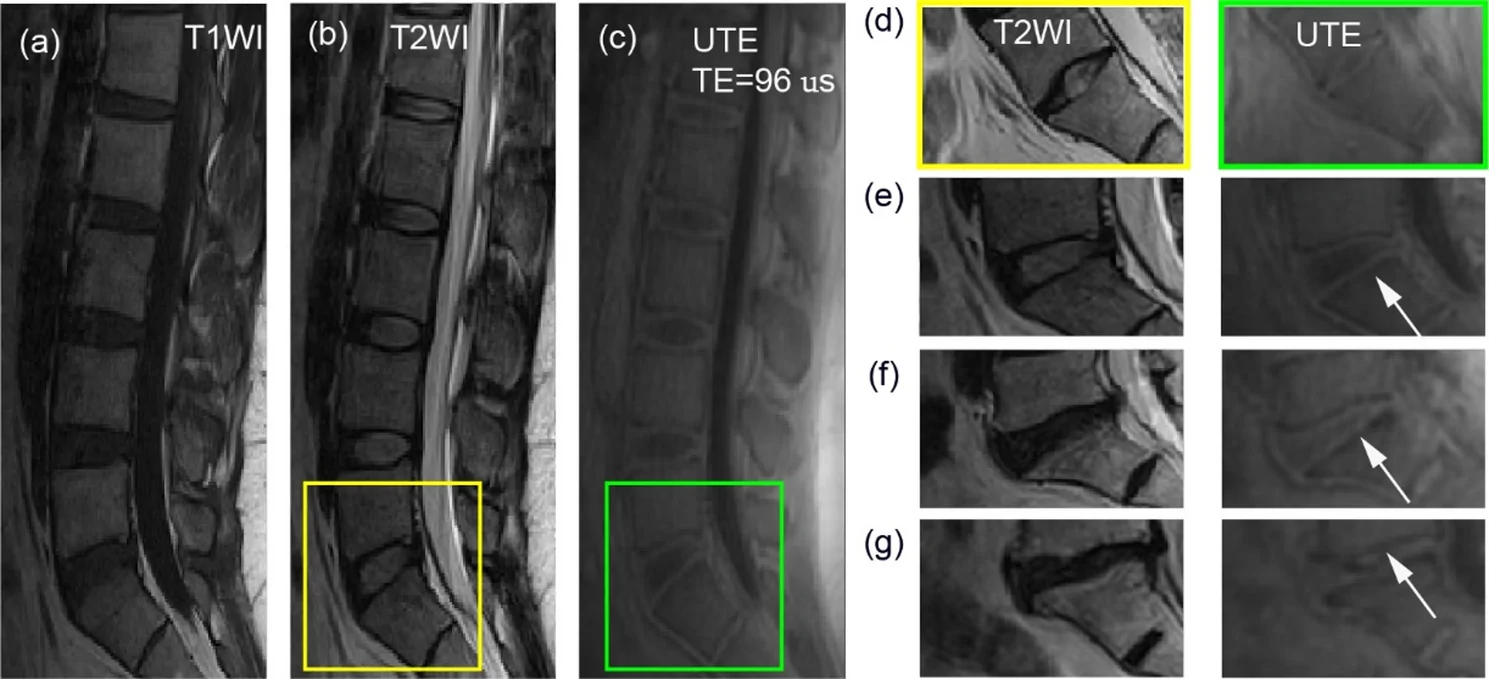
Comparison of Conventional versus Ultrashort Echo Time (UTE) Sequences in Lumbar Sagittal Magnetic Resonance Imaging of the Cartilage Endplate. The cartilage endplate signal is weak and difficult to recognize in (a) the T1-weighted image (T1WI) and (b) the $$\:{\mathrm{T}}_{2}$$-weighted image (T2WI). (c) Images obtained using UTE (TE = 96 ms) show the cartilaginous endplates of the lower lumbar segments with markedly high signals. Images (d–g) show the cartilaginous endplates in the L5–S1 region. The white arrowheads indicate areas of the endplates that are not visible in conventional sequences but show a high signal in UTE images. The left column is the T2WI image, and the right column is the corresponding UTE image
